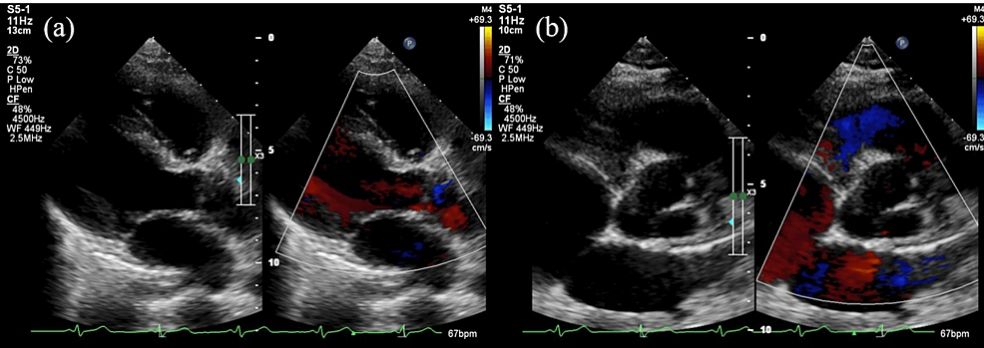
Transthoracic echocardiography at the end of treatment showing that the vegetation on the aortic valve is resolved but trivial aortic regurgitation remains (a) (b)
